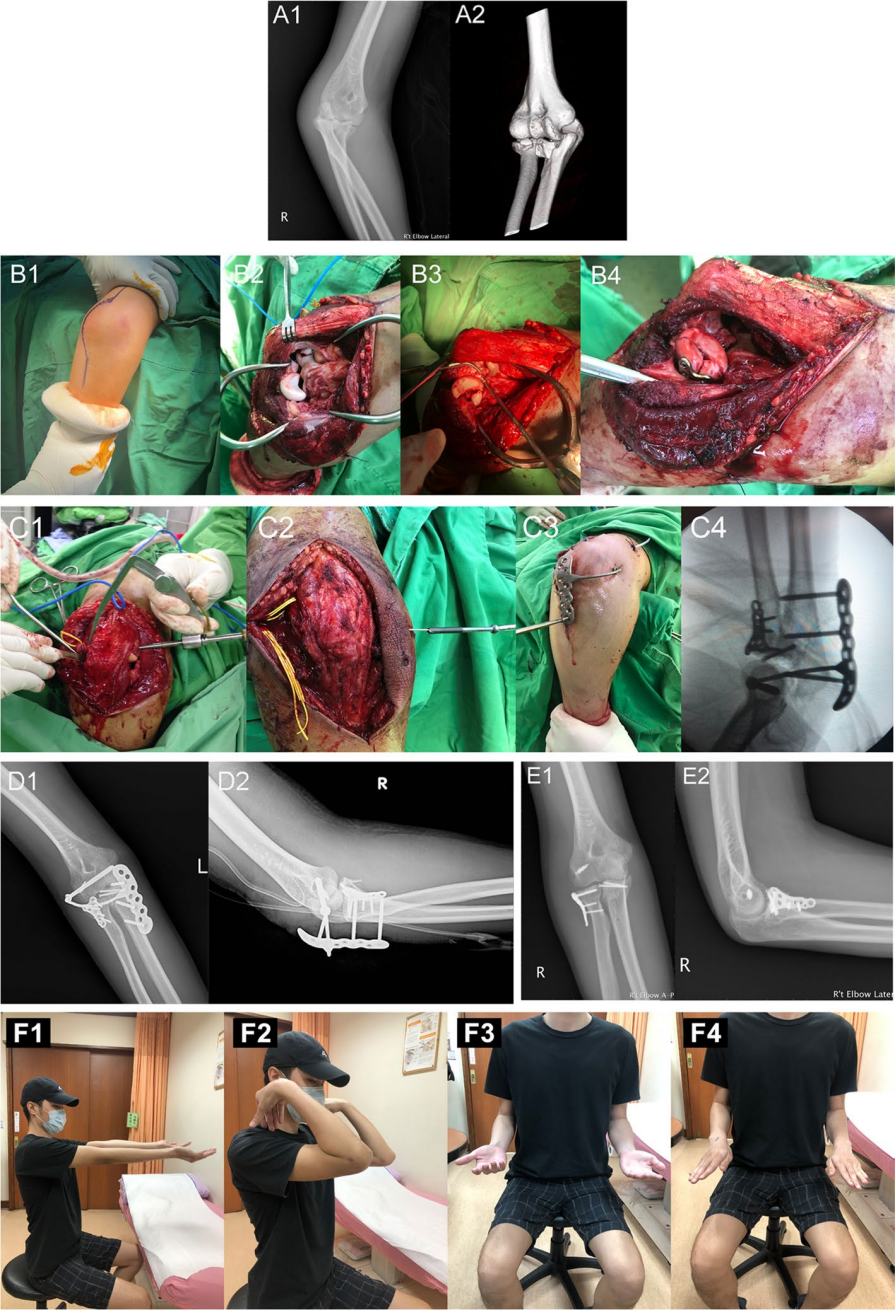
X-ray radiographs, computed tomography (CT) scans, and surgical procedure. (A1, A2) Radiograph and CT scan of a 29-year-old male patient with a right terrible triad of elbow dislocation (patient 16). The surgical photograph shows (B1) elbow exposure via a curved posterior incision, (B2) wide exposure of the elbow joint to reveal the lateral collateral ligament, radial head, and coronoid injury through the lateral or medial margin of the flaps, (B3, B4) radial head and coronoid process fractures treated using a plate and 2.4-mm cannulated screws for fixation. (C1) A line between the origins of both collateral ligaments reveals the axis; a 2.0-mm K-wire using an aiming device from the lateral side into the distal humerus along the axis, (C2) 5.0-mm cannulated screws inserted into the elbow rotational center, (C3) the hooked axis pin is inserted into the cannulated screw, and the external locking plate is fixed with 3.5-mm locking screws over the proximal ulna, (C4) stability in all directions was assessed under fluoroscopic guidance. (D1, D2) Anteroposterior lateral radiographs of the status after open reduction, internal fixation, lateral collateral ligament reattachment, and external joint stabilizer—elbow implantation. (E1, E2) Radiographic images of the elbow 1 year after the final removal of the implant. (F1-F4) Functional range of motion observed at the 1-year follow-up
